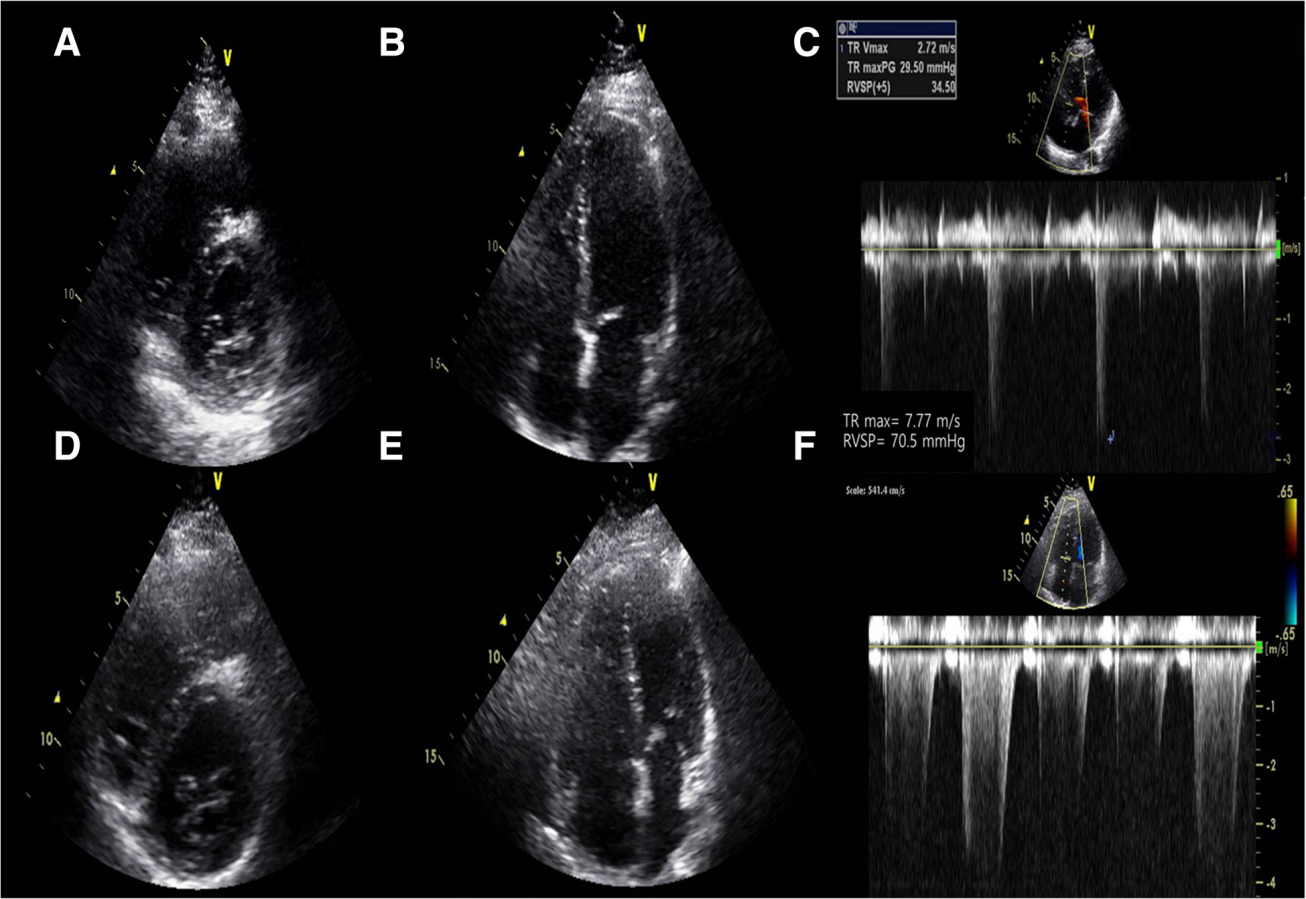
Changes of echocardiogrphic findings with 2.5 years of interval. Parasternal short axis (a) and apical 4-chamber (b) view at the initial visit demonstrated normal chamber size. Estimated RVSP was 34.5 mmHg (c). At the follow-up of 20 months, D-shaped LV (d) and RV enlargement (e) demonstrated estimated RVSP was 70.5 mmHg (f). LV; left ventricle: RV; right ventricle
